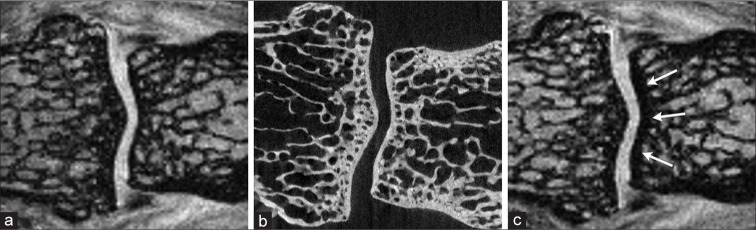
The subchondral bone thickness of this proximal interphalangeal joint was depicted similarly on the coronal (a) interleaved water-fat water + fat and (b) microcomputed tomography images. However, it appeared to be much thicker on the proximal side of the joint (arrows) on the (c) regular nonfat-suppressed gradient-echo image.
